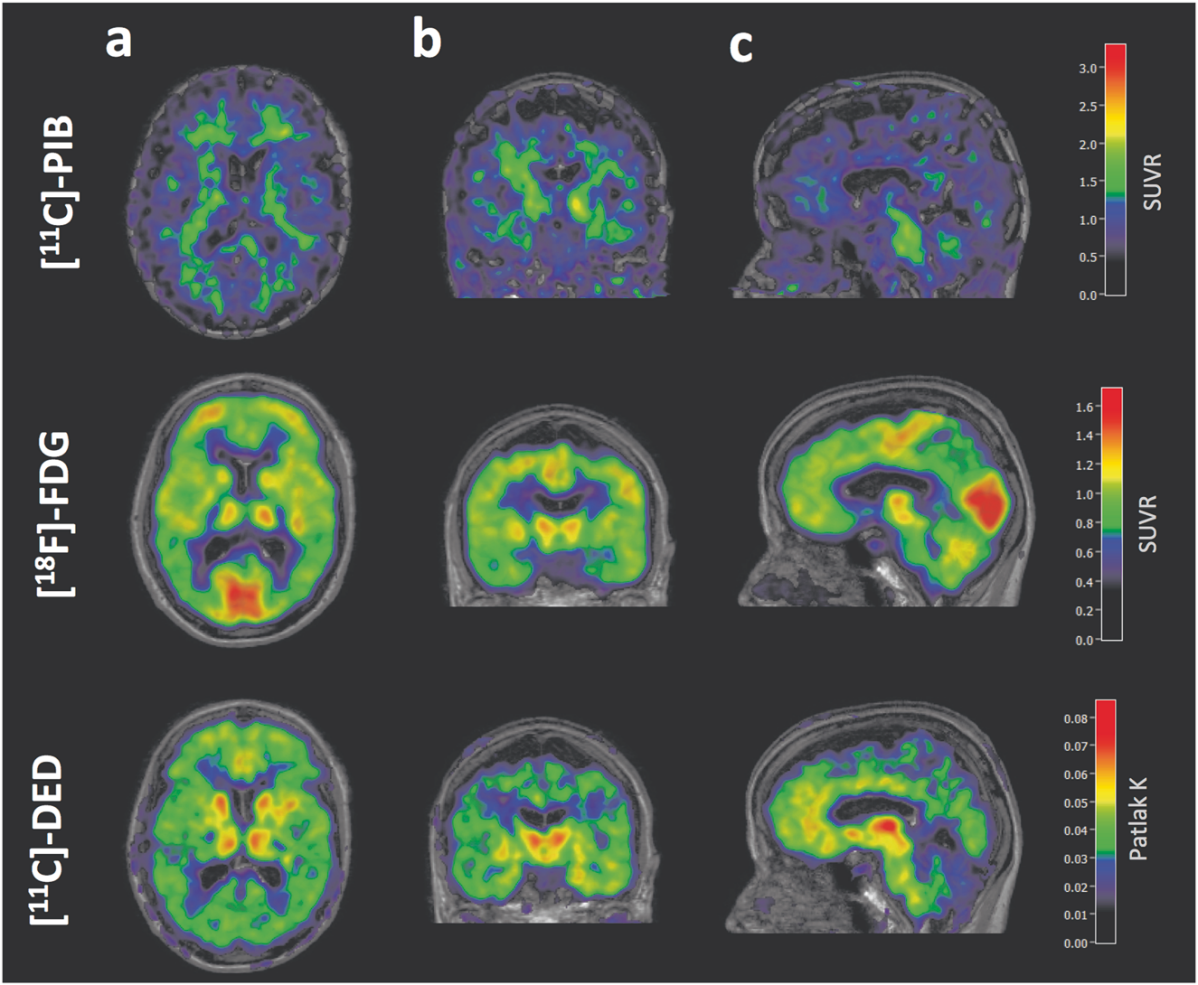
Multitracer PET scans for *AβPParc1* mutation carrier Multitracer PET scan for an *AβPParc* mutation carrier (*AβPParc1*) using ^11^C-Pittsburgh compound B (PIB), ^18^F-fludeoxyglucose (FDG), and ^11^C-deprenyl tracers. (^11^C-PIB and ^18^F-FDG late sum images (40–60 and 30–45 min, respectively) were created and co-registered onto the T1 MRI image. The whole pons was used as a reference for both tracers as it was found to be preserved from pathology in both autosomal-dominant and sporadic Alzheimer’s disease [14]. The ^11^C-deprenyl dynamic PET images were co-registered onto the T1 MRI image, and a modified reference Patlak model [15] was applied to the 20–60 min image using PMOD software, with the cerebellum as the modified reference region [16] to generate individual 3D parametric Patlak slope images (unit = min^−1^)). a horizontal section; b coronal section; c sagittal section.
